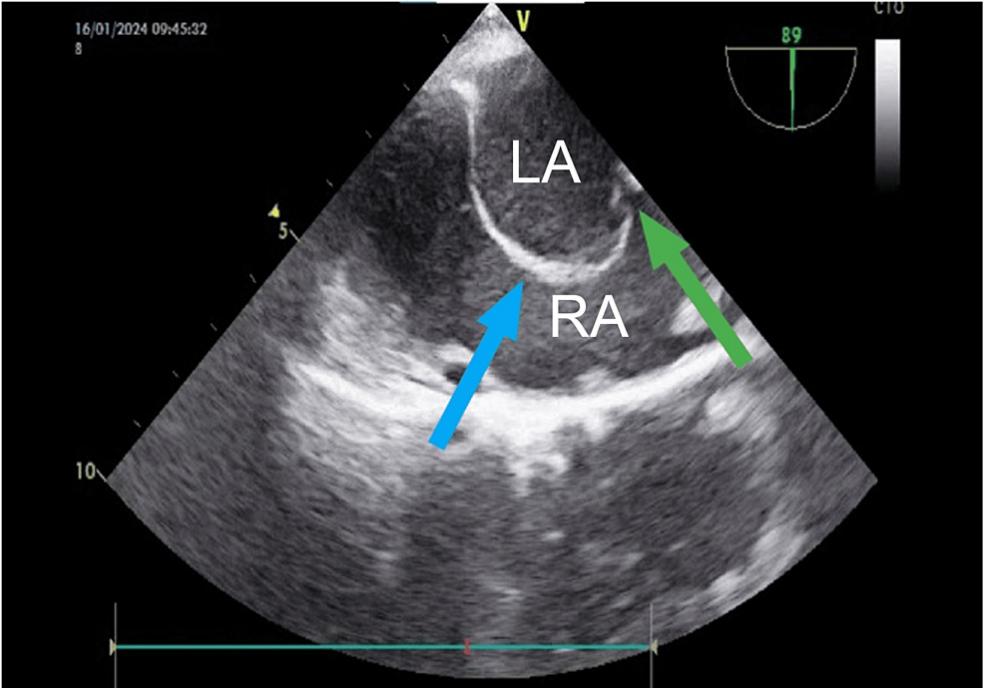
Transesophageal echocardiographic (TEE) image showing bulging atrial septum (blue arrow) and multiple fenestrations (green arrow). RA: right atrium; LA: left atrium.
